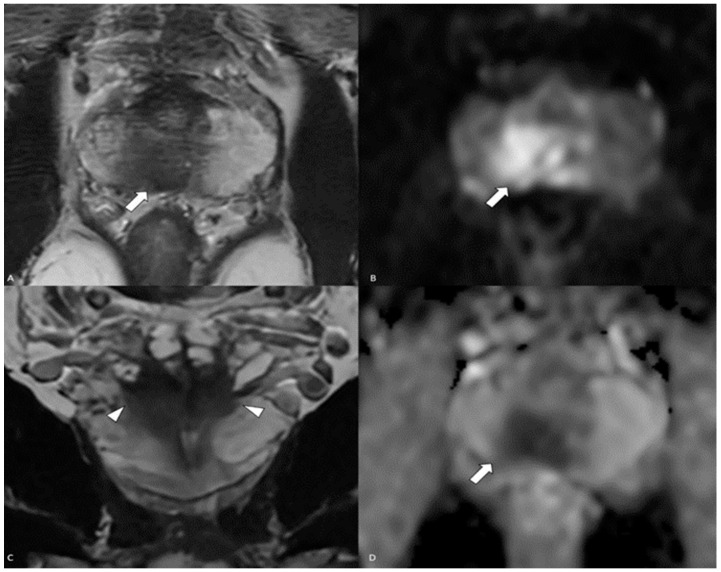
MRI of the prostate: (A) axial T2 demonstrates a posterior peripheral-zone T2-hypointense lesion (white arrow) with (B,D) restricted diffusion (white arrows), and (C) coronal T2 demonstrates seminal vesicle invasion (white arrowheads).
